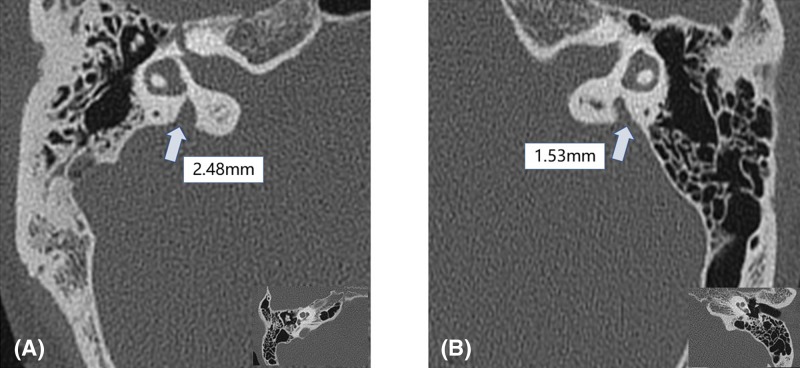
High-resolution temporal bone CT images of 2 cases with SLC26A4 mutation revealed IEVA Two cases ((A) patients XT148 and (B) XT089) of high-resolution temporal bone CT image; examples from 5 patients with IEVA in 63 patients with SLC26A4 mutation, based on the criteria of diameter greater than 1.5 mm between the outer of vestibular aqueduct and the vestibule of the total port foot or the midpoint of the isthmus.
